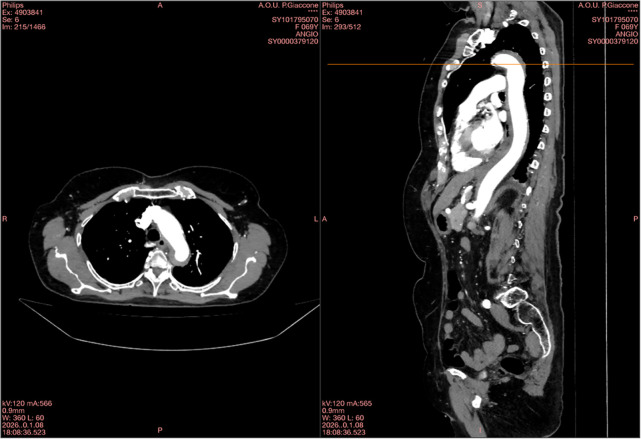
Initial CT angiography showing IMH extending from the mid aortic arch to the origin of the celiac trunk.
